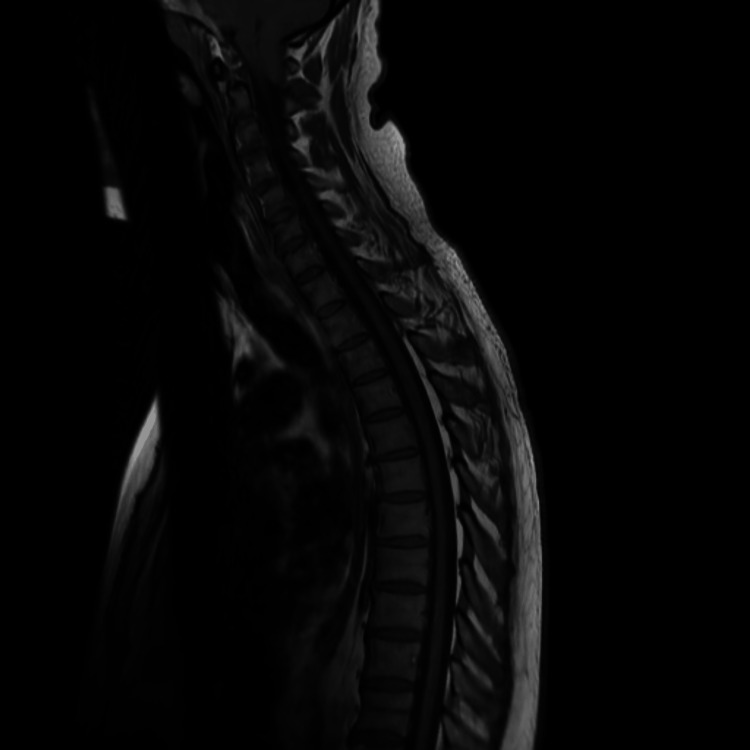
MRI whole spine showing no abnormalities
